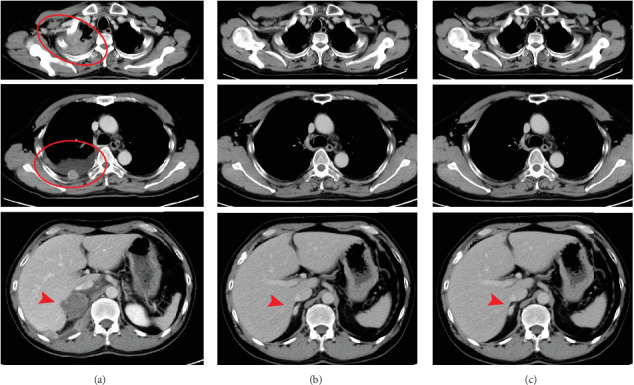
CT image (a) before ICI therapy and (b) after 9 months and (c) 20 months. The target lesions were widely spread as a large mass within the right pleura (circle) and adrenal gland (arrowhead) before therapy but are now localized to the metastatic site within the adrenal gland. CT, computed tomography; ICI, immune checkpoint inhibitor.
